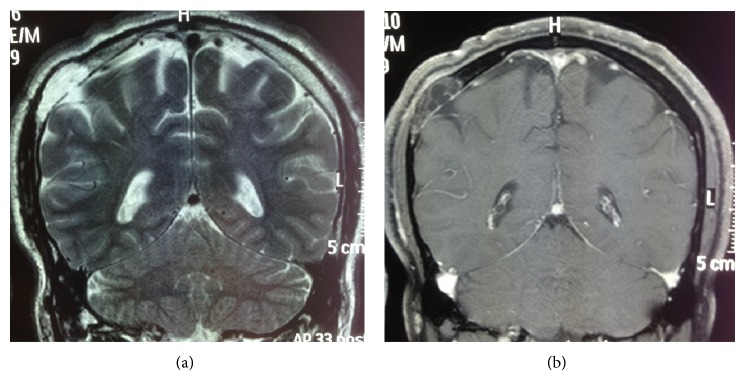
(a) T2 MRI showing high signal within the intraosseous lipomatous meningioma and dural defect. (b) T1 MRI with gadolinium observing heterogenous uptake within the lipomatous meningioma with no obvious intraparenchymal extension.
